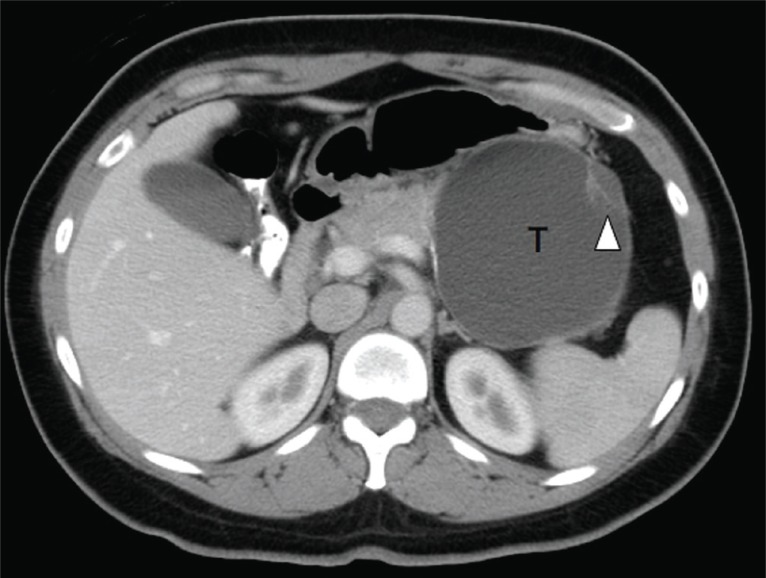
Computed tomography of the abdomen. This image shows a large cystic tumor in the left upper quadrant of the abdomen (indicated by T, right side of image). Arrowhead points to daughter locules.
